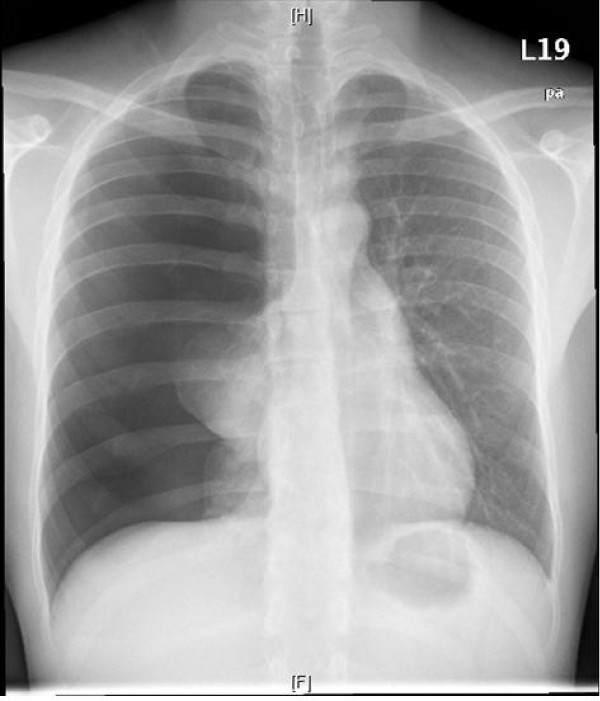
The first chest x-ray. We find a pneumothorax on the right with a subtotal lung collapse.
